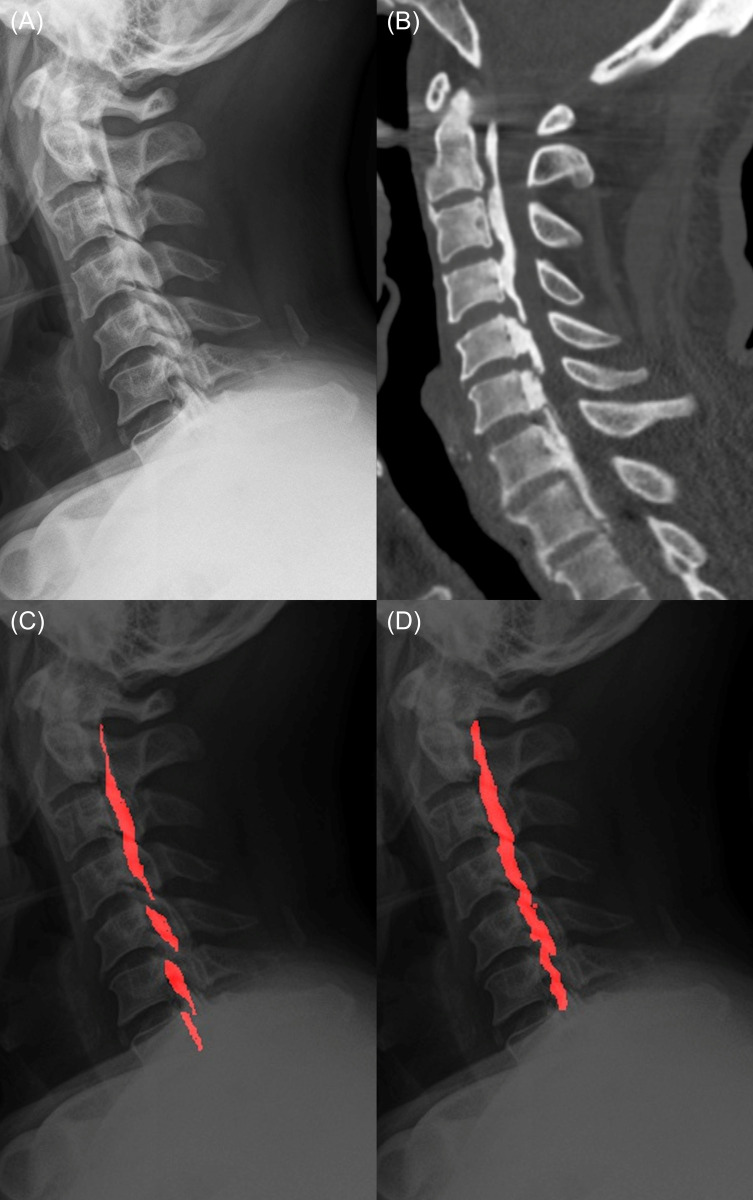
A 65-year-old male patient with mixed-type OPLL. (A) A lateral plain radiograph shows mixed-type OPLL along the posterior side of the vertebra. The lesion at the C7 level is obscured by the shoulder shadow. (b) In the sagittal image of cervical spine CT, ossifications ranging from the C2 to T1 level are clearly demonstrated (window width = 2000 HU, window level = 500 HU). (c) OPLL lesions annotated by a radiologist on the plain radiograph. (d) In the resulting image inferred by the deep-learning model, OPLL lesions at the C2-6 levels are well predicted, but the lesion located at the C7 level was not detected by the model. OPLL, ossification of the posterior longitudinal ligament.
